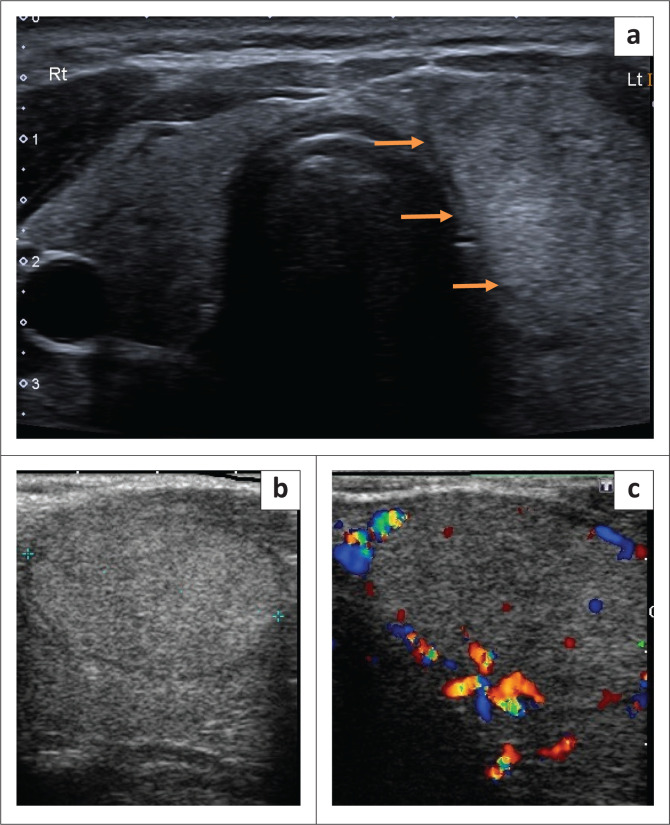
(a) Transverse view ultrasound image of the thyroid gland shows a well-defined, isoechoic, oval nodule with a thin peripheral halo (orange arrows) in the left lobe. (b and c) Longitudinal view ultrasound. The lesion in the left lobe of the thyroid gland (b) demonstrates predominantly peripheral flow on colour flow Doppler (c).
